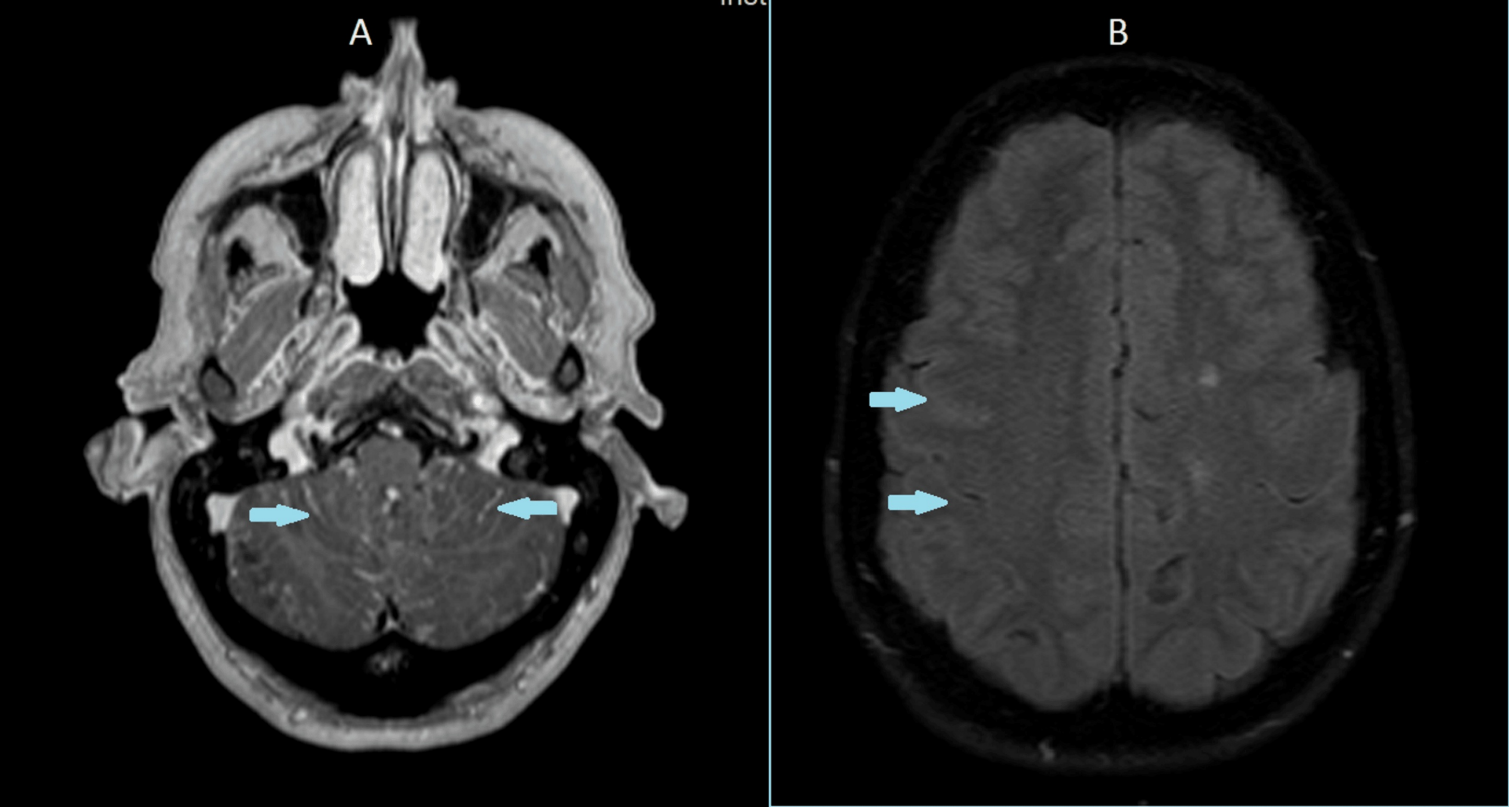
Contrast-enhanced brain magnetic resonance imaging demonstrated bilateral cortical and sulcal FLAIR hyperintensity with leptomeningeal enhancement involving the cerebral hemispheres, cerebellum, and brainstem, without nodular enhancement-findings compatible with an inflammatory or infectious meningeal process. A subtle periventricular hyperintensity suggestive of transependymal edema was also noted (A: Axial plane (posterior fossa); B: Axial plane (supratentorial/cerebral hemispheres)) FLAIR: Fluid-attenuated inversion recovery
